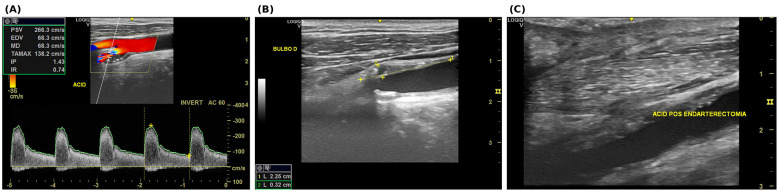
Carotid duplex ultrasonography of a patient with 70%−99% stenosis (NASCET) of the right internal carotid artery (RICA). (A) Color Doppler and spectral analysis of the RICA demonstrating an elevated peak systolic velocity (PSV) of 266.3 cm/s and end-diastolic velocity (EDV) of 68.3 cm/s, with PSV exceeding the CREST-2 cut-off (≥230 cm/s) for high-grade stenosis. (B) B-mode ultrasonography of the right carotid bulb (BULBO D) prior to carotid endarterectomy (CEA), showing heterogeneous atherosclerotic plaque (plaque length 2.25 cm; maximal thickness 0.32 cm). (C) B-mode ultrasonography of the RICA after CEA (“ACID pós endarterectomia”), demonstrating a patent arterial lumen without significant residual stenosis. Source: authors' personal archive.
